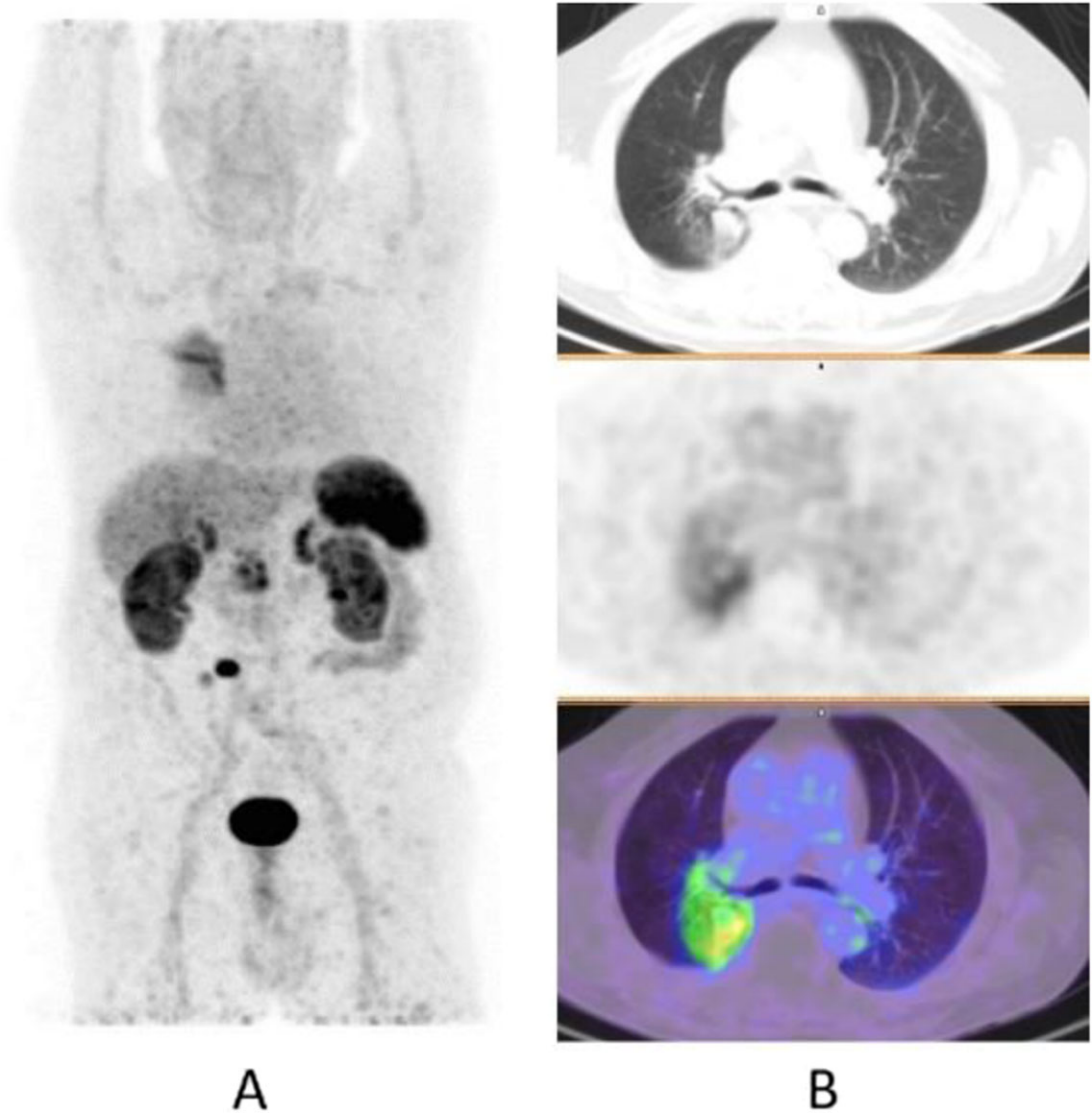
^68^Ga-DOTA peptide, follow-up of midgut NET, specificity. *Clinical history*: 69 y.o. woman with NET treated by surgery the year before. Right upper lobe lung adenocarcinoma treated by chemo- and radiation therapy 4 years prior. *PET/CT findings*: in addition to multifocal recurrent disease (a MIP), ^68^Ga-DOTANOC PET shows moderately increased uptake in the previously irradiated lung parenchyma (b)
